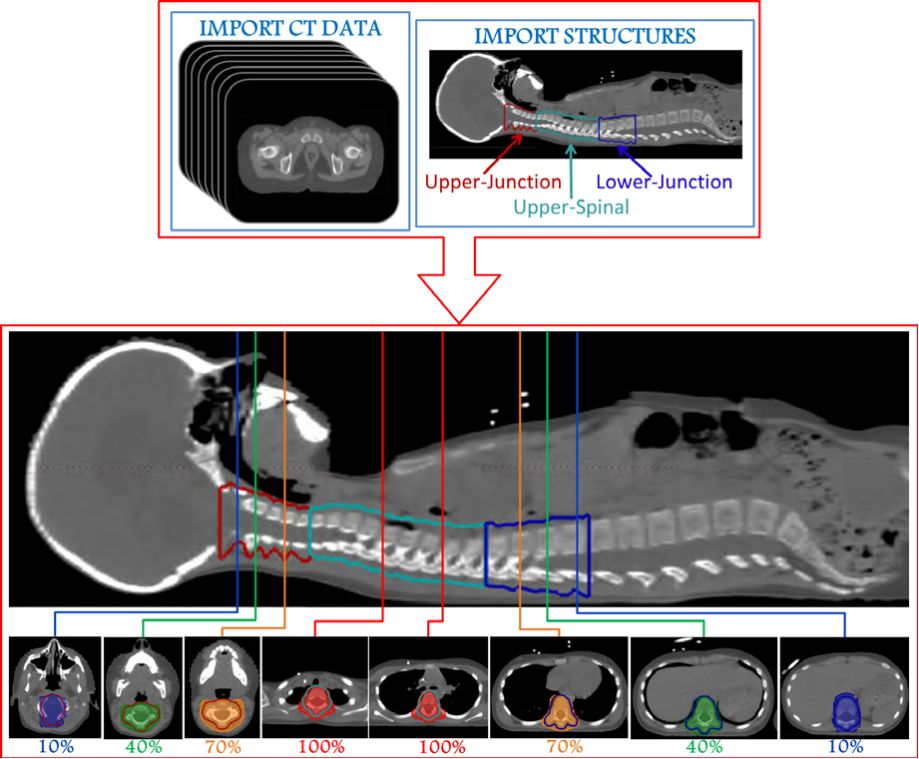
Background dose generation. Patient CT data and structures are exported from the TPS and uploaded into a numerical computing environment (MATLAB
